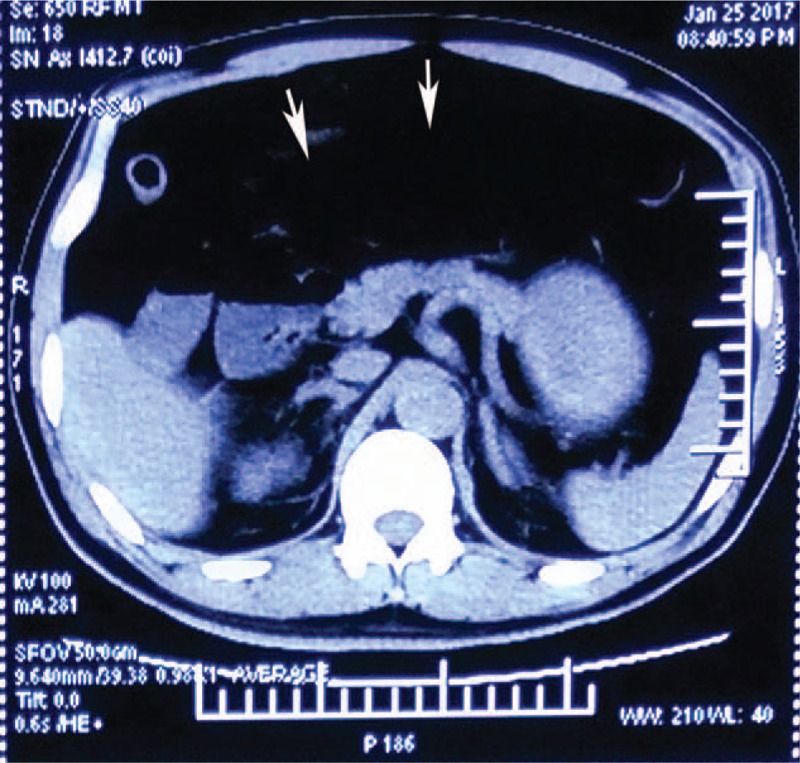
Computed tomography of the abdomen reveals that the small bowel was dilated (the white arrows).
